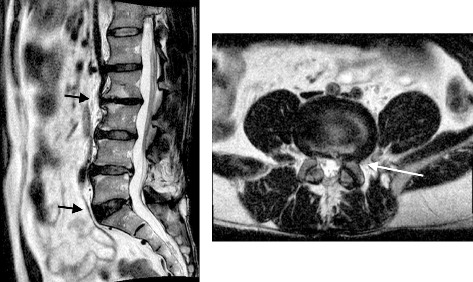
Lumbar spine magnetic resonance images show dehydrated lumbar discs with a loss of normal T2 signal intensity (black arrows). A diffuse disc bulge is seen indenting the anterior aspect of the thecal sac at L4-5 (white arrow).
